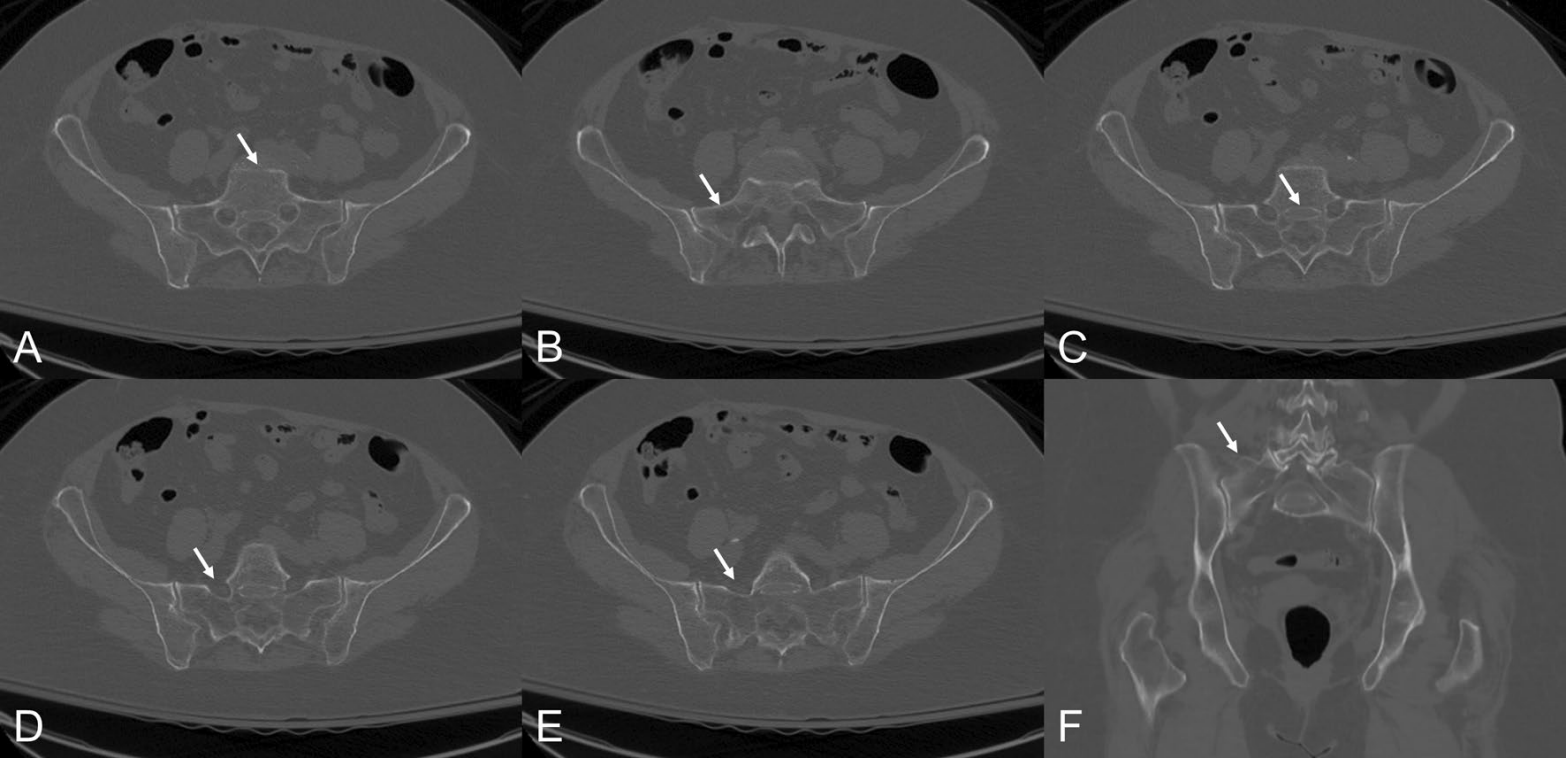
CT images showing dysmorphic features. Axial images demonstrate: (a) S1 not recessed in the pelvis, (b) acute alar slope, (c) residual disk, and (d, e) misshapen sacral foramina. Coronal imaging demonstrates (f) mammillary bodies
